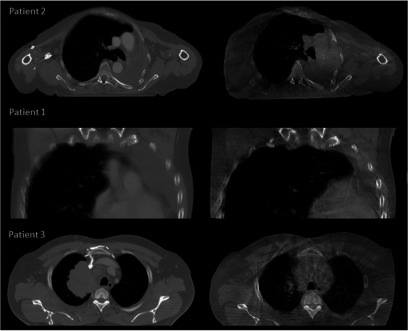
Examples of minor (Patient 2), moderate (Patient 1) and major (Patient 3) anatomical changes. For each patient the pCT is shown on the left and the CBCT is shown on the right.
